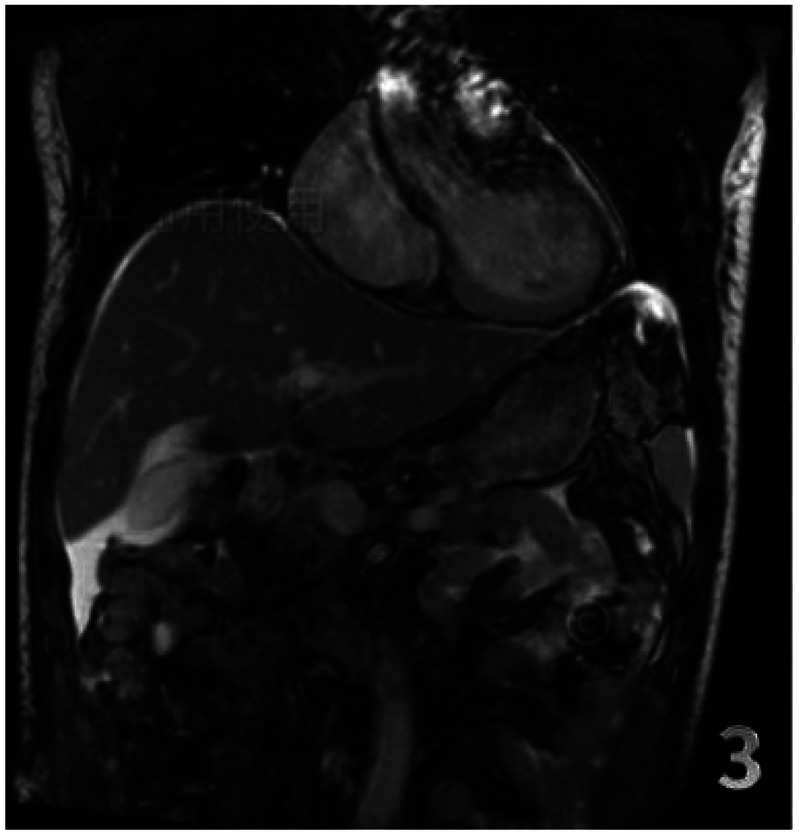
MRI showed pericholecystic fluid collection.
